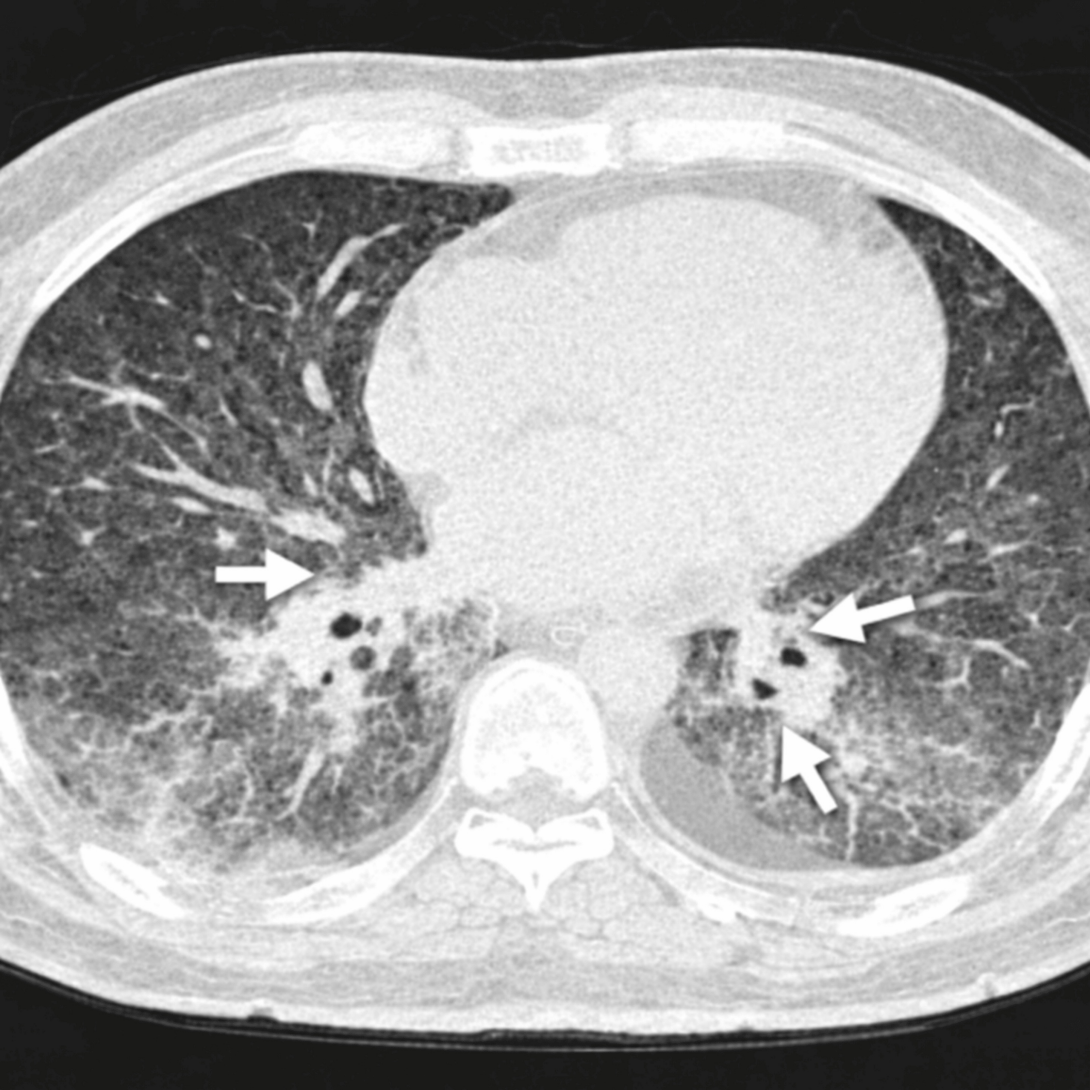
Chest CT showing bilateral posterior basal ground-glass opacities with areas of consolidation.
